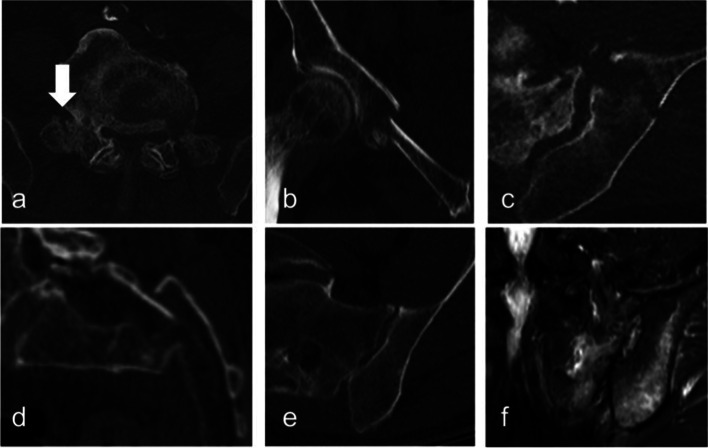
Three modifiers of the OF-Pelvis classifications system for osteoporotic sacral and pelvic ring fractures. Modifier can be assigned alone or in combination and shall indicate more severe injury a) Modifier 1 fracture of the L5 transverse process b-d) Displacement at any localization e) CT shows only a fracture of the sacral ala and f) the MRI reveals additional edema in the iliac bone without fracture evidence in the CT
